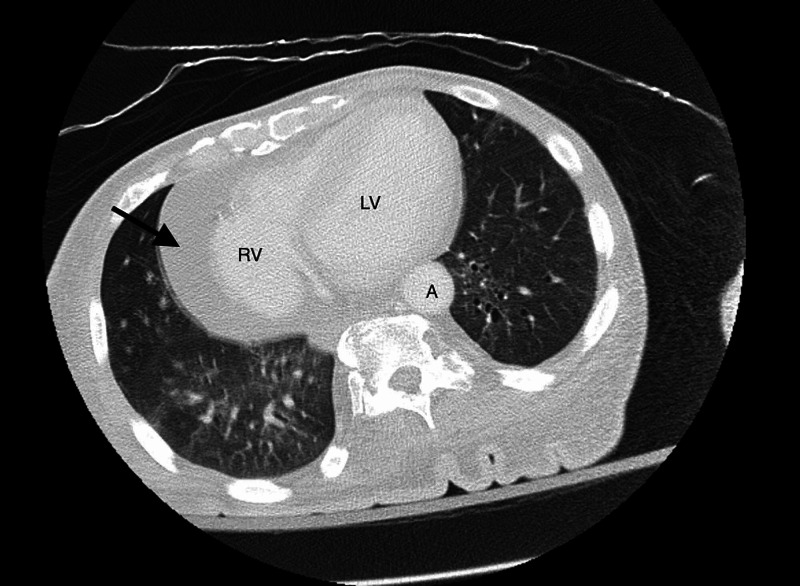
CT chest: moderate- to large-sized pericardial effusion Black arrow showing pericardial effusion; RV: right ventricle; LV: left ventricle; A: aorta; CT: computed tomography.
